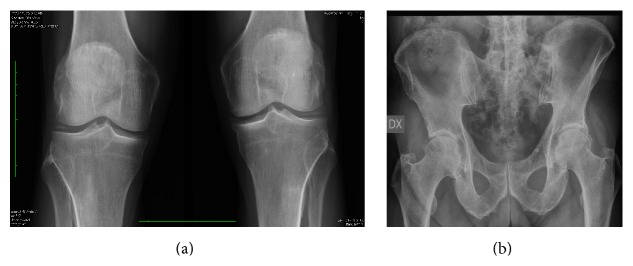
Conventional X-ray of knees (a) and hips (b). The figure shows a picture of osteoarthritis associated with calcifications in menisci (a) and in the soft tissues near the hip bilaterally (b).
